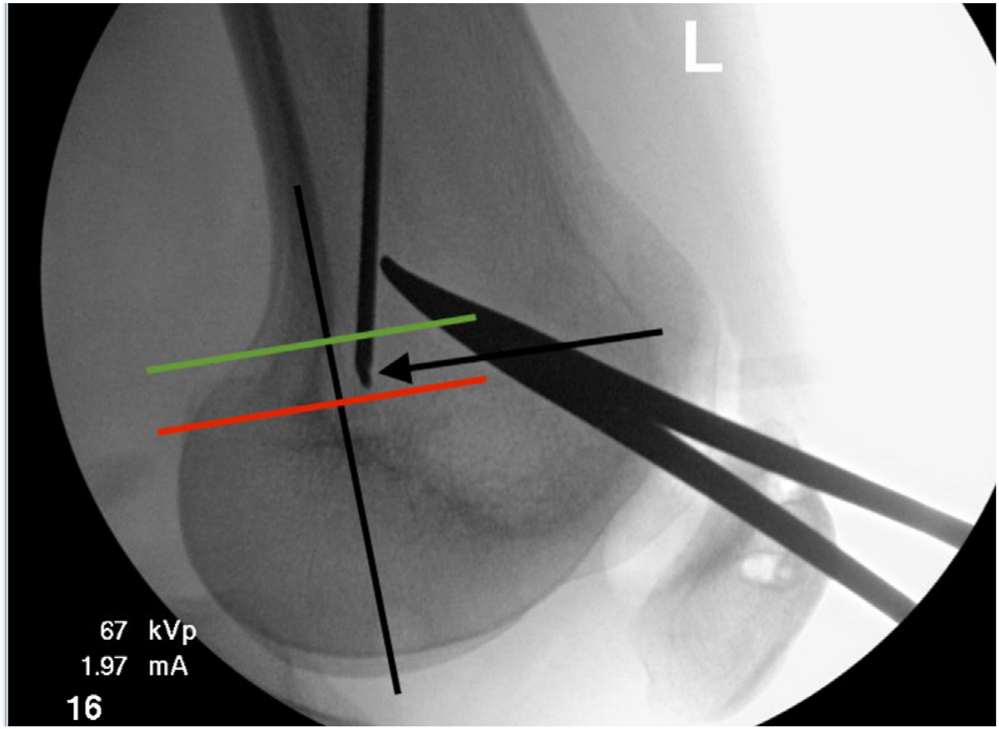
The rendering is of a left knee with the patient in the supine position. The patient's head would be to the right side of the image out of view and the foot would be to the left side of the image. Intraoperative identification of Schottle’s point (arrow). A perfect lateral x-ray is obtained. Schottle’s point is identified just anterior to a line tangent to the posterior femoral cortex (black line) and between horizontal lines between the posterior aspect of Blumensaat’s line (red line) and the superior aspect of the femoral condyle (green line).
